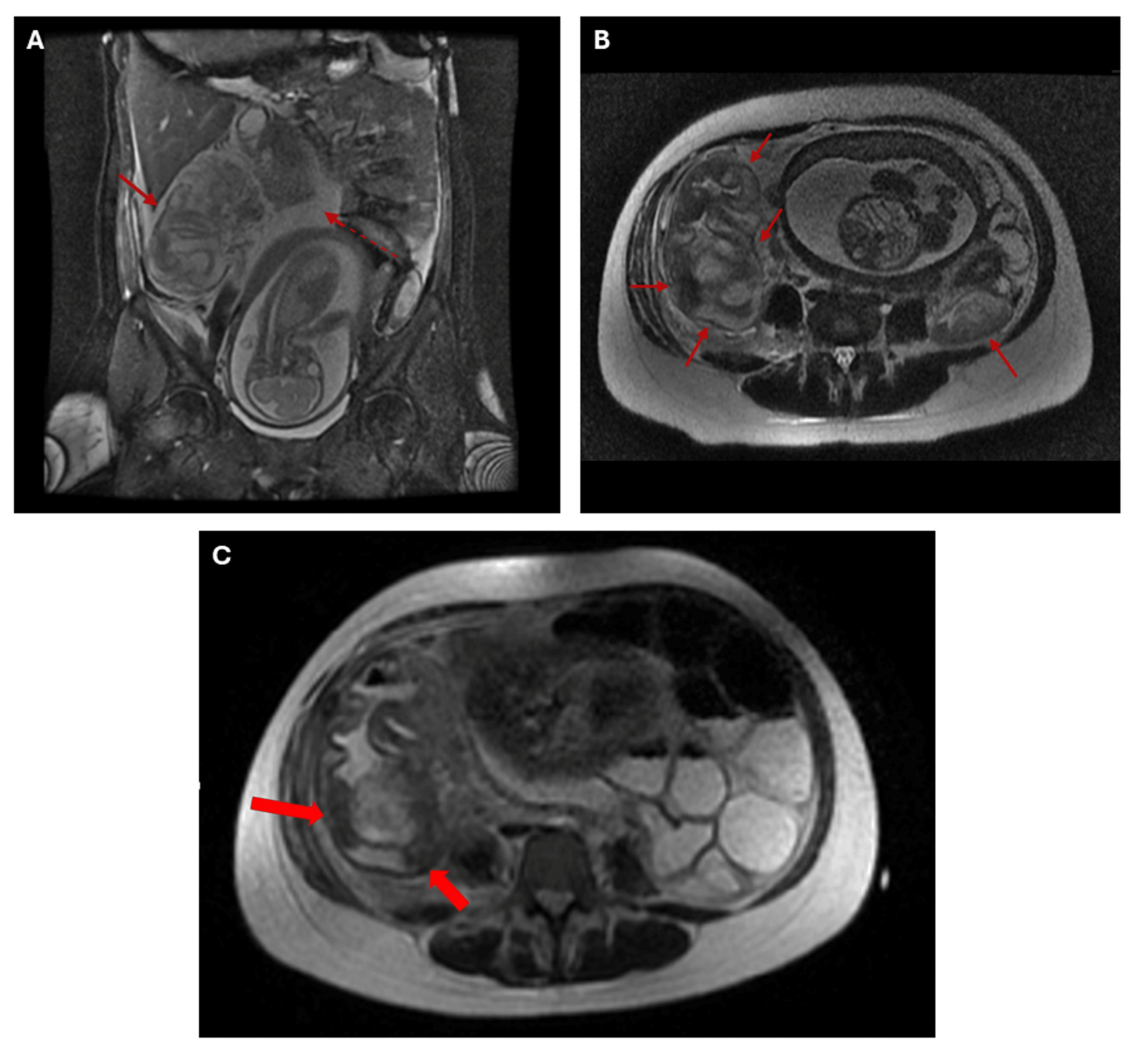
Coronal and axial abdominal MRI. A. Coronal FIESTA FS: Concentric, regular thickening of the submucosa of the cecum and ascending colon with areas of hypointense T2 signal (red arrows), suggestive of colitis of probable infectious versus inflammatory etiology, with associated submucosal hemorrhage. Free fluid is also noted (red dashed arrow).
